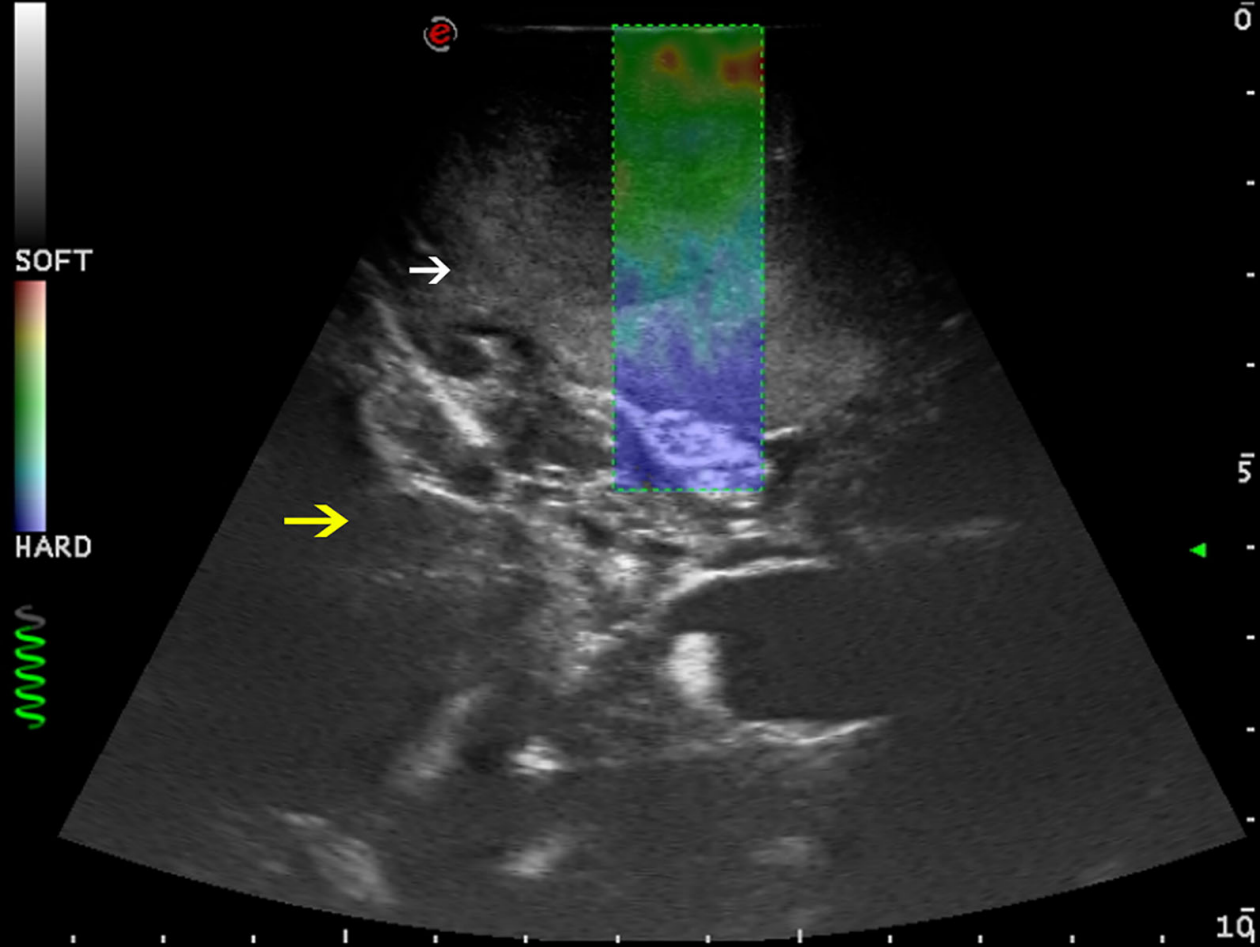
Strain elastosonography frame in a case of right parietal GBM (case no. 3). Elastosonography gives information about the stiffness of the tissue, and it is revealed through a chromatic scale. Red spots are representative of softer zones (necrotic areas); blue spots are representative of harder zones (brain–tumor interface and brain parenchyma). The core of the lesions appears to be softer than surrounding normal brain parenchyma. White arrow: GBM. Yellow arrow: surrounding normal brain parenchyma.
